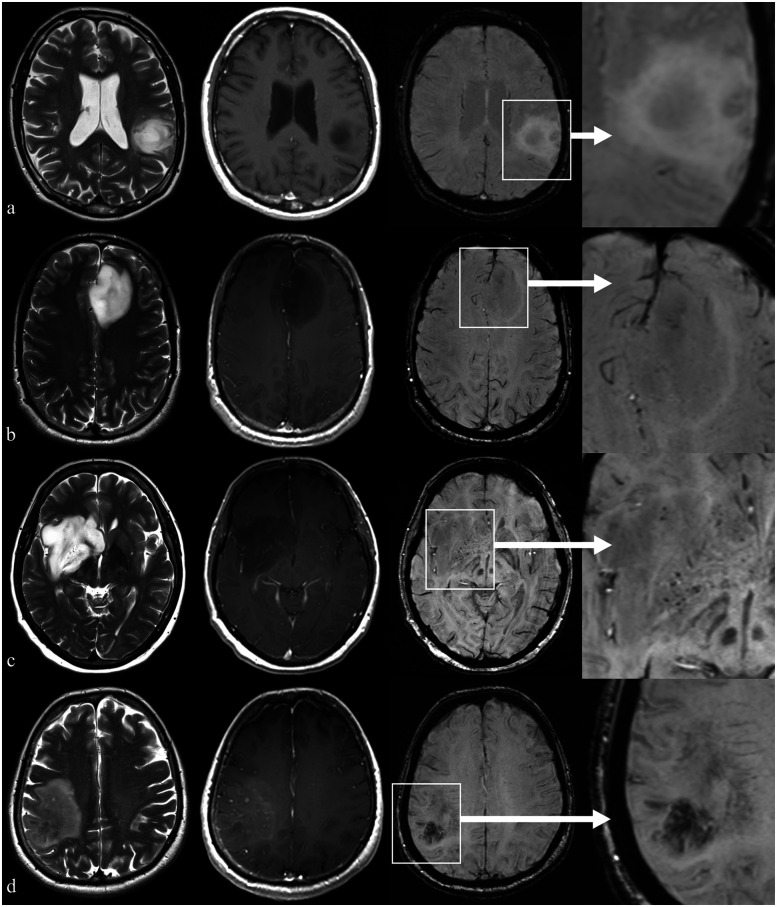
Example of patients with brain glioma presenting with different ITSS grades but similar findings on conventional imaging. First column: axial T2-weighted images; second column: axial T1-weighted images acquired after i.v. injection of gadolinium-based contrast medium; third column: axial 3D susceptibility-weighted images (SWI); last column: a magnified view of the area of interest on SWI. Row (a) parieto-temporal glioma infiltrating the left supramarginal and angular gyri in a 47 y.o. right-handed male showing no relevant findings on SWI (ITSS grade 0); row (b) left frontal glioma infiltrating the anterior cingulate gyrus in a 36 y.o. male with a cluster of (<5) tiny hypointense black dots in SWI (ITSS grade 1); row (c) right basal ganglia and insular glioma in a 55 y.o. female showing multiple (<10) millimetric intralesional hypointense spots on SWI (ITSS grade 2); row (d) right parietal glioma glioma in a 72 y.o. male showing diffuse hypointense subcortical deposits on SWI (ITSS grade 3).
